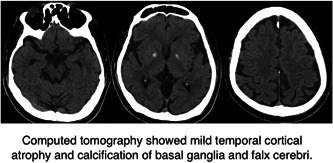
Computed tomography showed mild temporal cortical atrophy and calcification of basal ganglia and falx cerebri.
